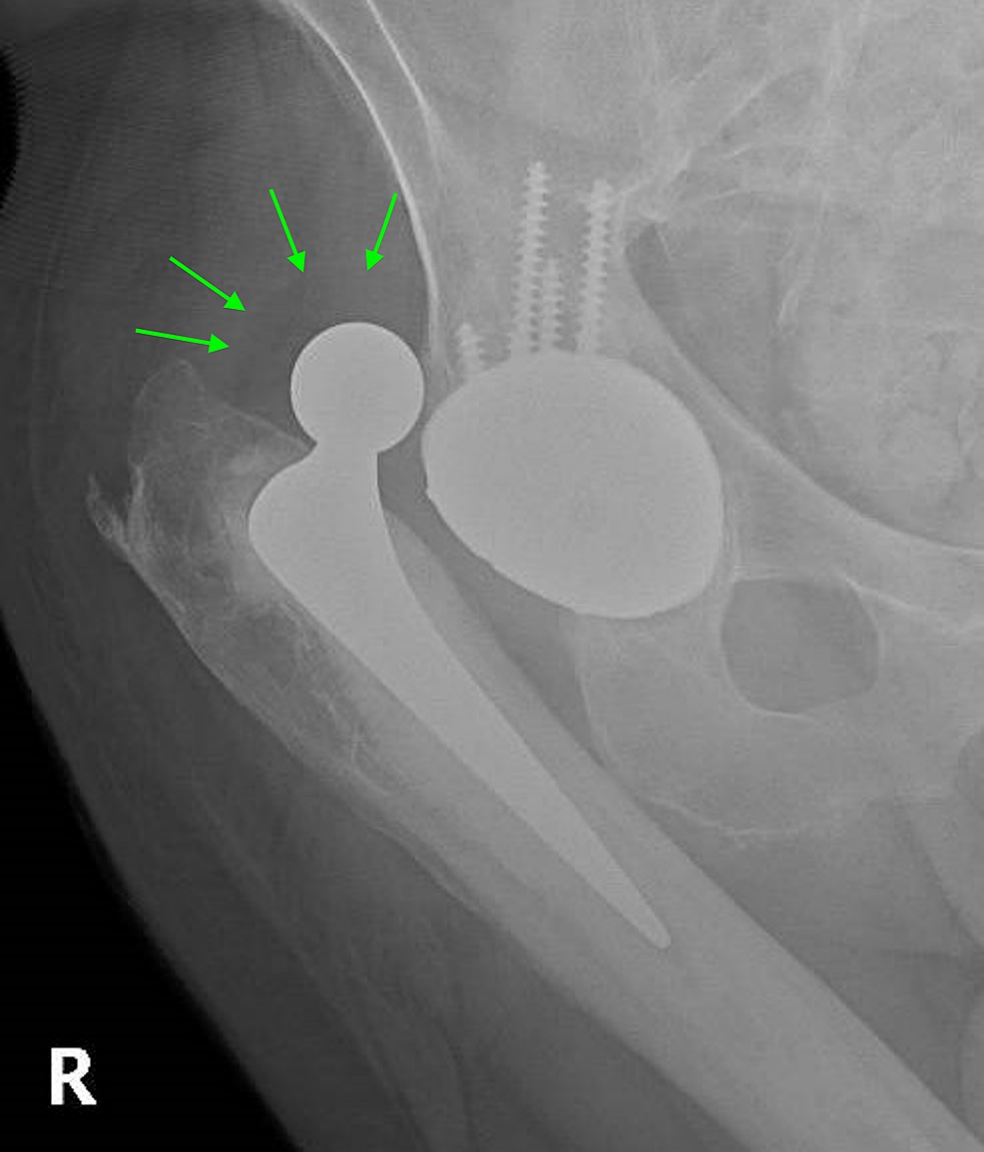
Anteroposterior X-ray of the right hip showing dislocated polyethylene liner-metal cup articulation of dual-mobility total hip arthroplasty. The polyethylene liner is attached to the prosthetic head, as demonstrated by the green arrows.
